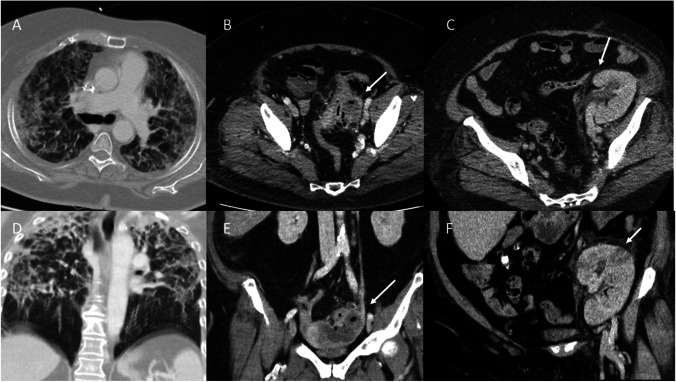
Imaging examples of patients with sepsis who received diagnostic CT. Patient 1 was a 62-year-old female with infiltrates confined to the upper lobes of both lungs consistent with a diagnosis of pneumonia (a axial 5-mm reconstruction with lung kernel, d coronal plane); this patient died during hospitalization. Patient 2 was a 61-year-old female with a fluid-collection in contact both with the colon sigmoideum and the left adnexa; the imaging differentials included both an abscess due to perforated diverticulitis and a tubo-ovarian abscess (b axial 5 mm with soft tissue kernel, e coronal 5 mm), with the latter being confirmed by surgery. Patient 3 was a 69-year-old male with peripheral perfusion deficits and perirenal fluid (arrows) in a kidney transplant (c axial 5 mm with soft tissue kernel, f coronal 5 mm)
